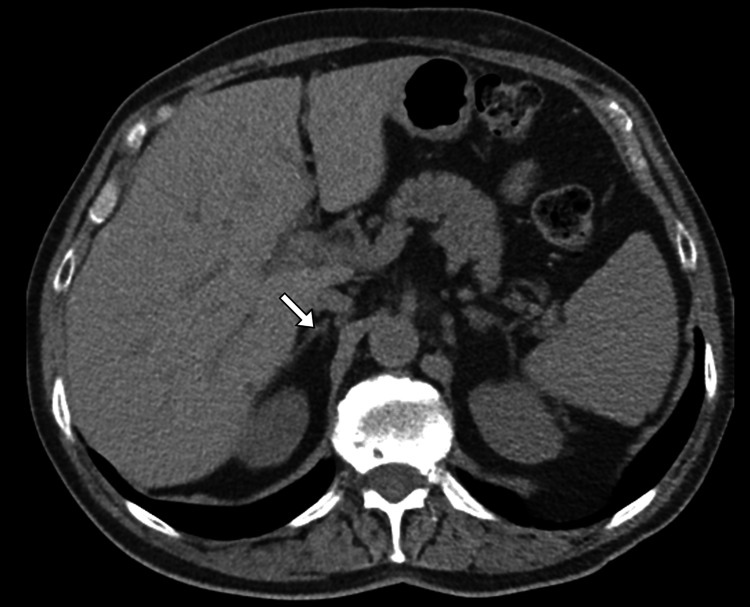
Interval resolution of right adrenal hematoma on follow-up CT Follow-up non-contrast axial image of the abdomen demonstrates near-complete resolution of the previously identified right adrenal hematoma (arrow), with no residual mass or new adrenal abnormality CT: computed tomography
